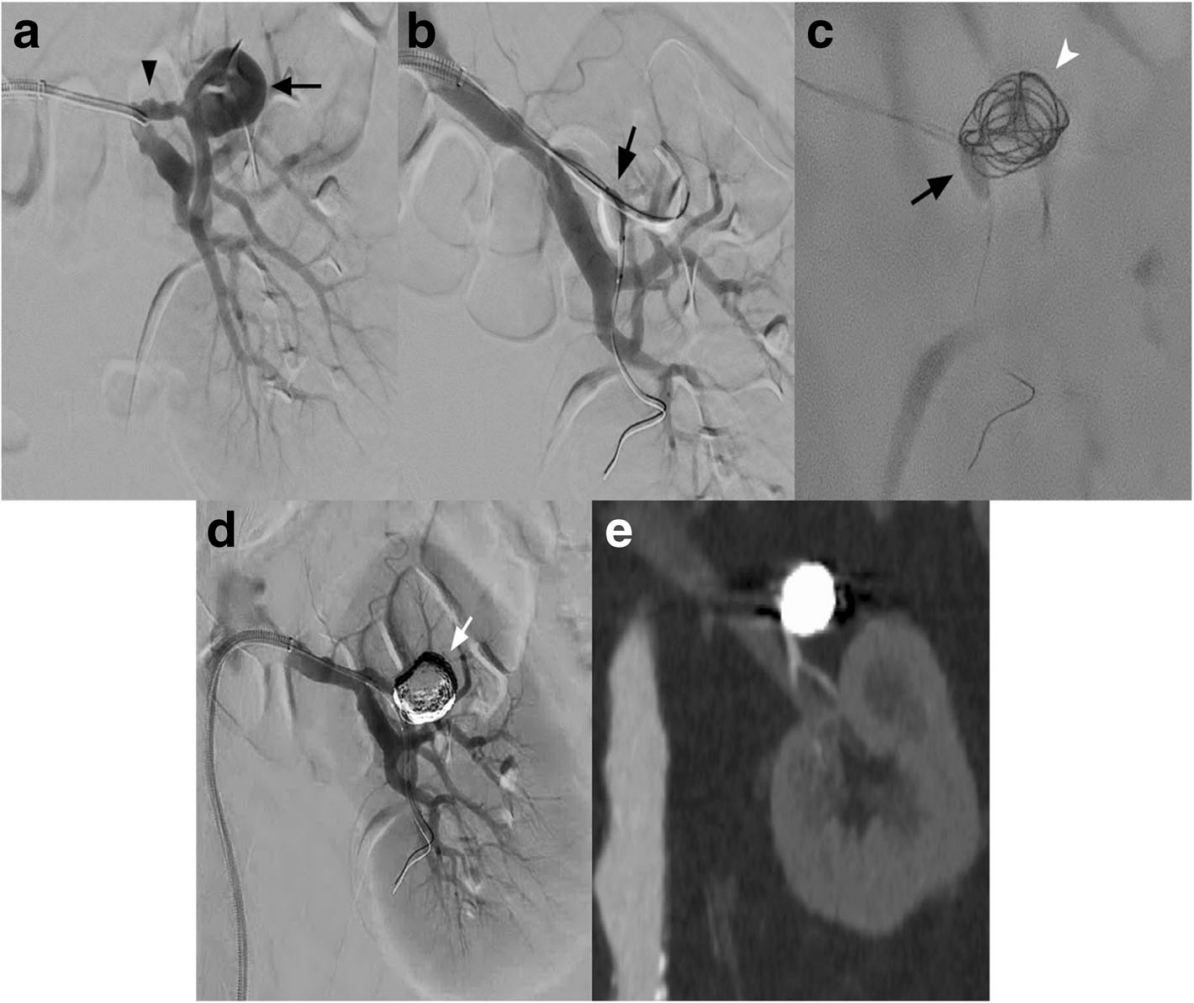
a Selective angiography of the left renal artery anterior division confirming the presence of a 2 cm wide-necked saccular aneurysm (arrow). The parent renal artery demonstrates a beaded appearance suggestive of fibromuscular dysplasia (arrow head). b Re-modelling of the wide-necked TRAA using the Scepter C balloon (arrow) which is inflated across the aneurysm neck. The coiling microcatheter tip has been positioned within the aneurysm sac. Angiography through the guide sheath shows occlusion of flow within the aneurysm sac and distal anterior division branch. c Fluoroscopic image demonstrating the inflated Scepter C balloon (arrow) with delivery of the initial detachable coil within aneurysm sac (arrow head). d Final completion angiography demonstrating complete obliteration of the aneurysm sac by a dense coil ball (arrow) with preservation of flow into the distal anterior division branch and renal parenchyma. e Follow-up CT angiogram at 3 months demonstrating persistent occlusion of the TRAA sac with normal solitary kidney parenchymal enhancement
